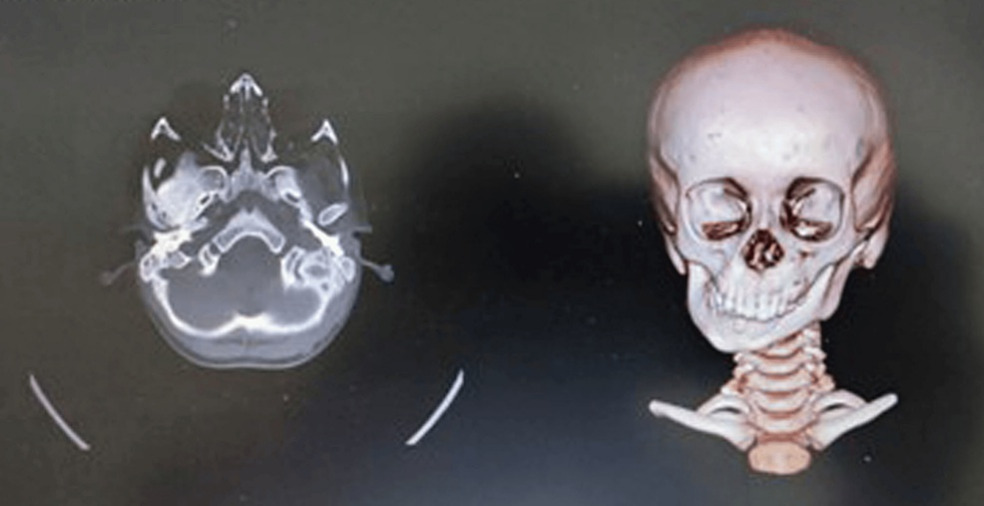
CT and 3D reconstruction of head confirming TMJ ankylosis. TMJ: temporomandibular joint.
